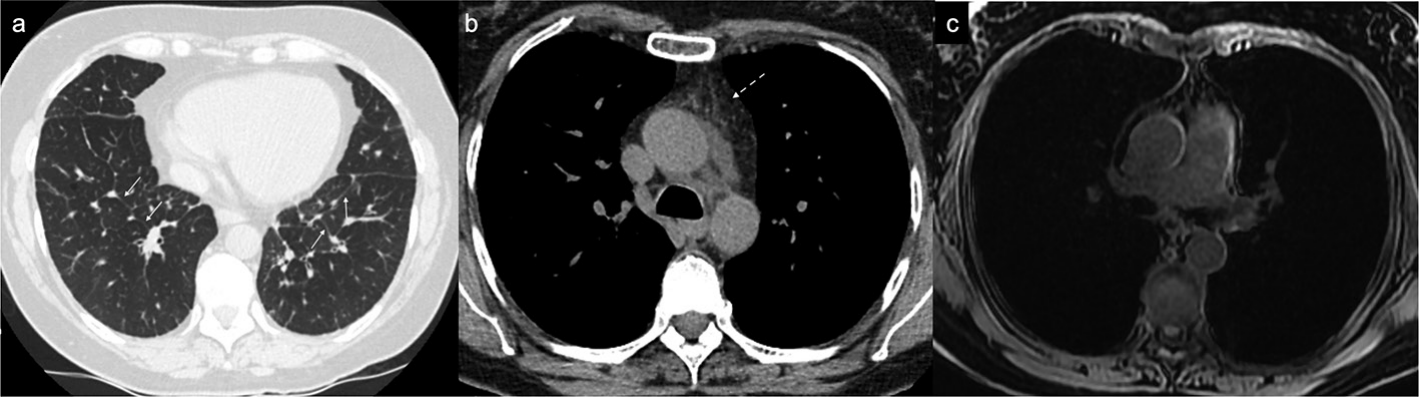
Patient present with dyspnoea and chest CT findings of mediastinal fat stranding (white broken arrow) and bilateral smooth interlobular septal thickening (white arrow) (Figure 6a–b), and raising possibility of pulmonary lymphangiomatosis. DCE MR lymphangiogram showed no abnormal intra thoracic lymphatic vessels (Figure 6c). DCE, dynamic contrast-enhanced; MR, magnetic resonance.
